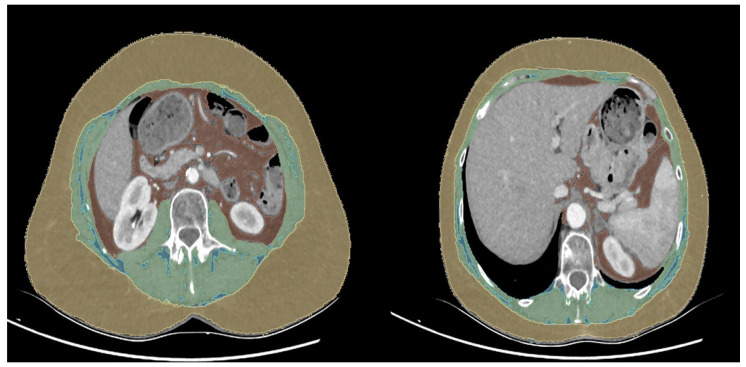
Computed tomography-based body composition analysis at the third lumbar vertebra (L3, left panel) and the twelfth thoracic vertebra (T12, right panel) levels.
